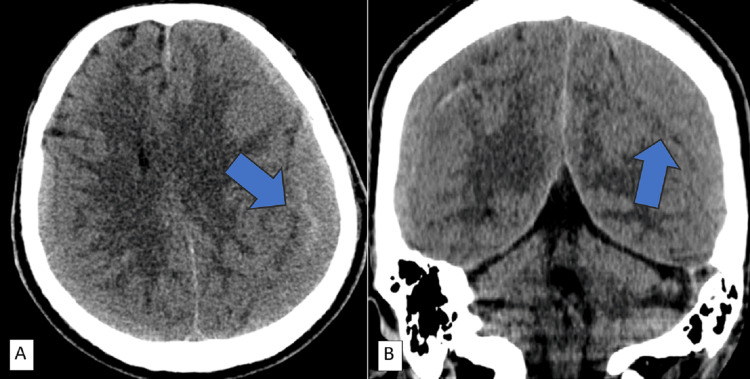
Non-contrast enhanced CT brain Axial (A) and coronal (B) views show bilateral subdural hyperdense collections in the frontoparietal regions (blue arrows).
